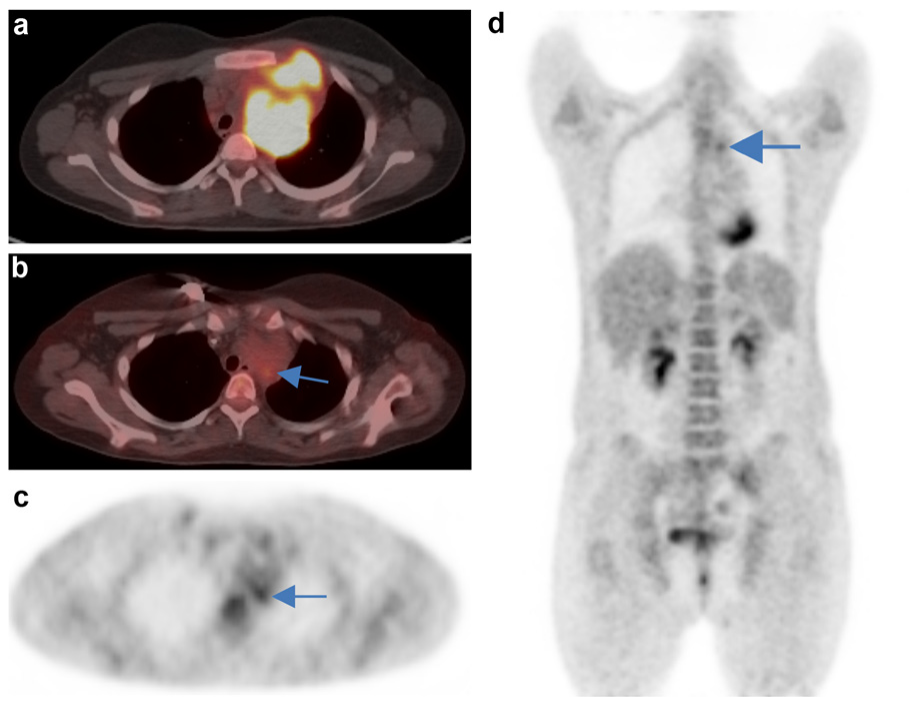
Discrepant D scoring of the mediastinum. Case 39: a) extensive lymphoma involvement of the left side of the upper mediastinum before treatment. b) residual mass after two cycles ChT. c) highest residual FDG uptake in the dorsal part of the residuum. D) It is difficult to decide if this highest FDG uptake is above or below the liver uptake. The interpretation is complicated by the small size of the hottest part of the residuum. Deauville score of the readers R1-5, respectively: 3-3-4-4-4. Courtesy M. Reinhardt, Dept. Nuclear Medicine, Pius-Hospital, Oldenburg, Germany.
